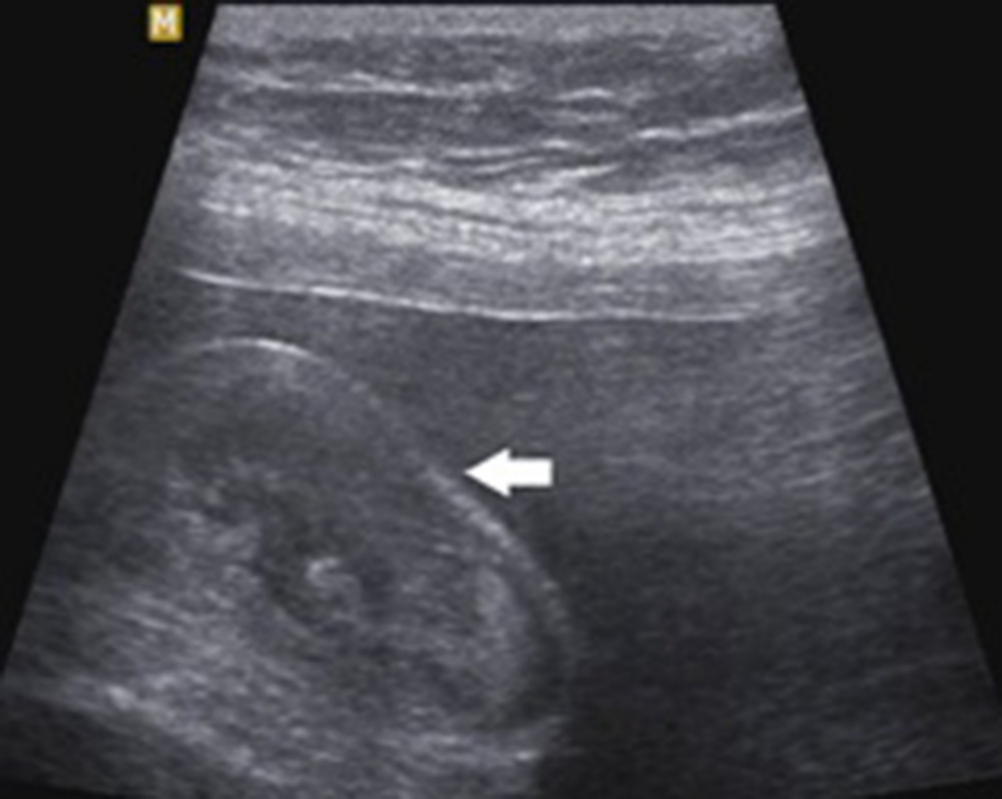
Longitudinal sonography of foetal scrotum showing concave surface (arrow).
